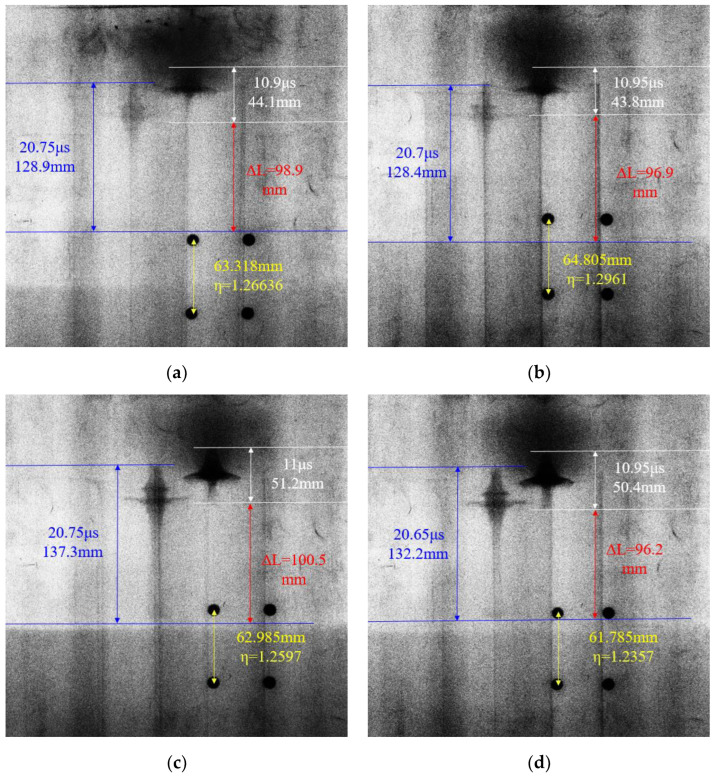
Pulse X-ray shooting test results: (a) PLA-1#. (b) PLA-2#. (c) PLA-Cu-1#. (d) PLA-Cu-2#.
